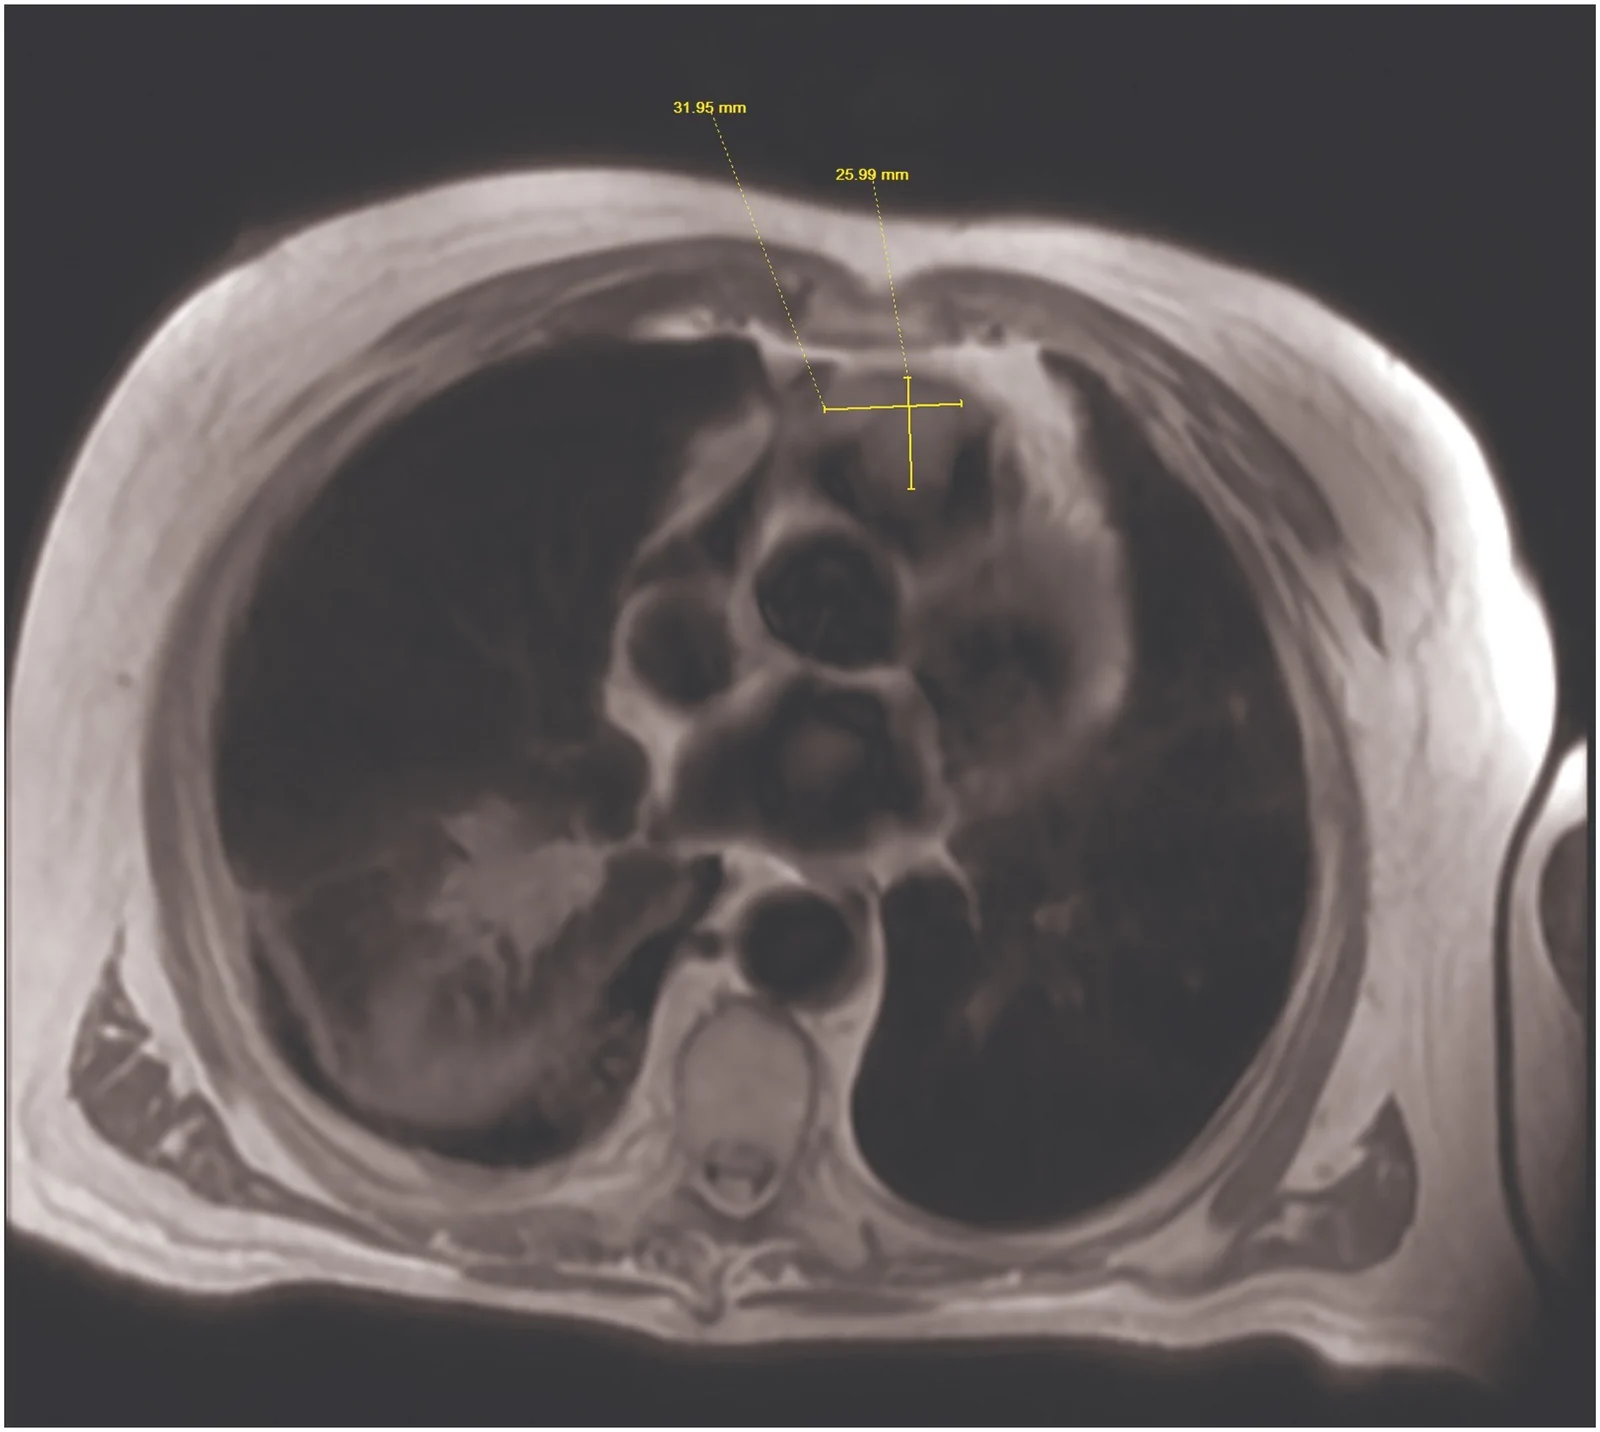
Cardiac magnetic resonance imaging. Demonstrating a lobulated mass (arrows) along the anterior right ventricular free wall, extending into the right ventricular lumen proximal to the pulmonic valve.
